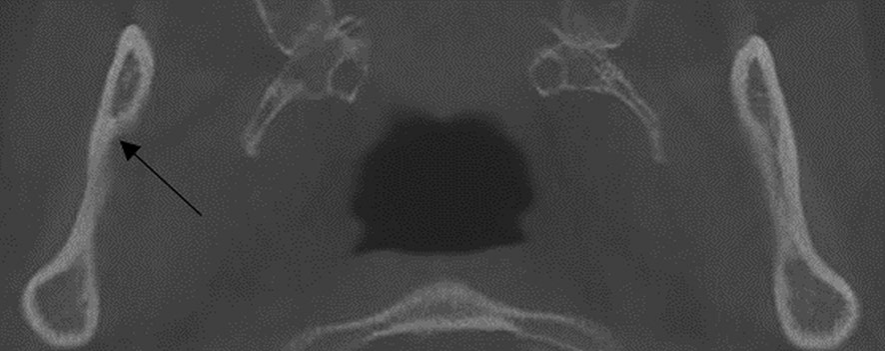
Confirmation of MSD presence in CBCT axial section.
